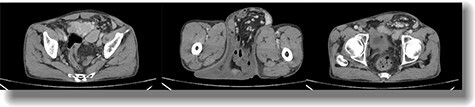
Axial postcontrast CT of the abdomen at the level of pelvis showing a defect in the peritoneal lining in the left inguinal region with protrusion of bowel loops, accompanying mesentery and vessels into the left scrotal sac via inguinal canal suggestive of left inguinoscrotal hernia.
